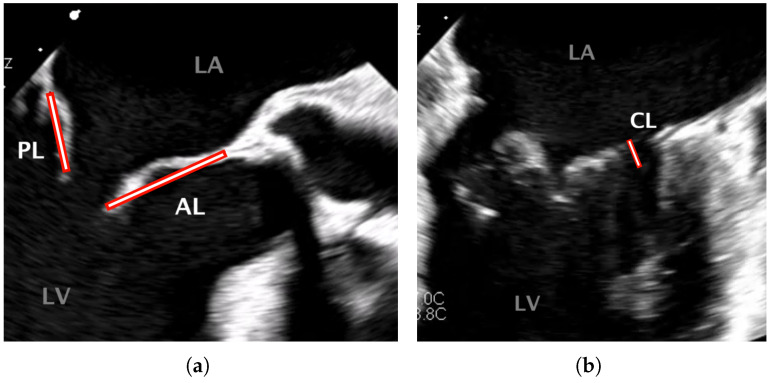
Parameters used to construct the geometry of the mitral valve leaflets, shown in an ultrasound image. LA: Left atrium. LV: Left ventricle. (a) Anterior leaflet (AL) and posterior leaflet (PL) length measurements. (b) Commissural length (CL) measurement.
